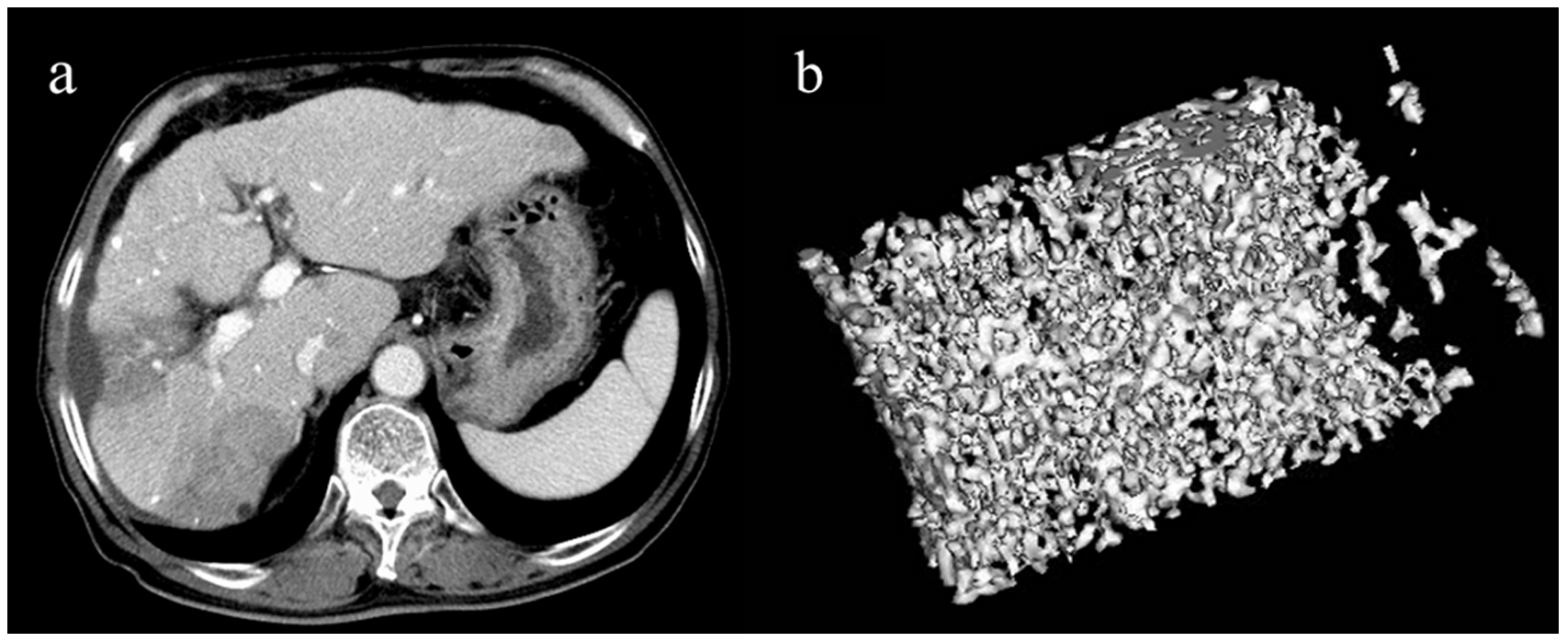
Representative 3D MDCT images of the L3 vertebra obtained from a 71-year-old man after 24 dynamic CT scans and seven transarterial chemoembolization (TACE) procedures for hepatocellular carcinomas. An axial CT image of the liver shows segmental low density areas in the S5 and S7 areas due to previous TACE procedures(a). The 3D image shows sparse trabecular bones (b) compared to those of the patient in Figure 3. Tissue bone mineral density (36.2 mg/cm^3^) is lower than the mean value of control subjects. The image is cut in half along the longitudinal midline.
